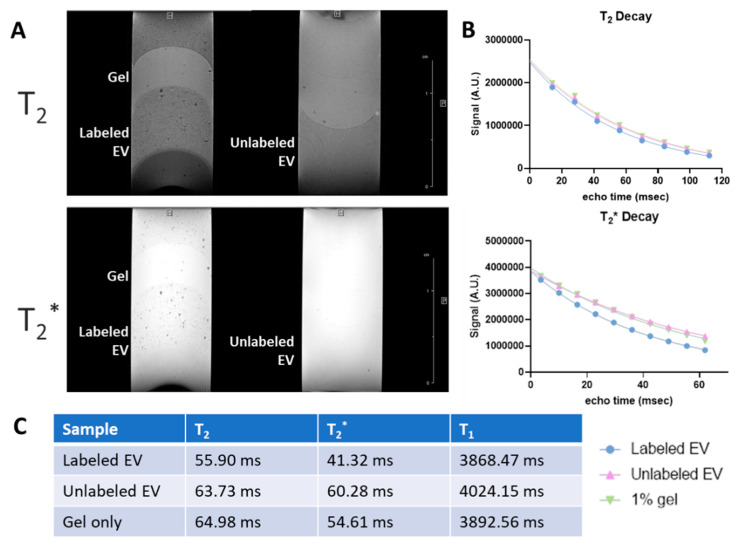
In vitro MRI for labeled brain organoid−EVs (15–20 nm). EVs sonicated with nanoscale iron oxides, 6 cycles: 30 s on/30 s off. Layered in agarose gel; (A) Preliminary results show the contrast of EVs containing iron oxides in MR images. (B) T_2_ and T_2_^*^ decay rates are demonstrated for labeled and unlabeled EV compared to gel only. (C) T_2_, T_2_^*^, and T_1_ values were extracted from their respective plots.
